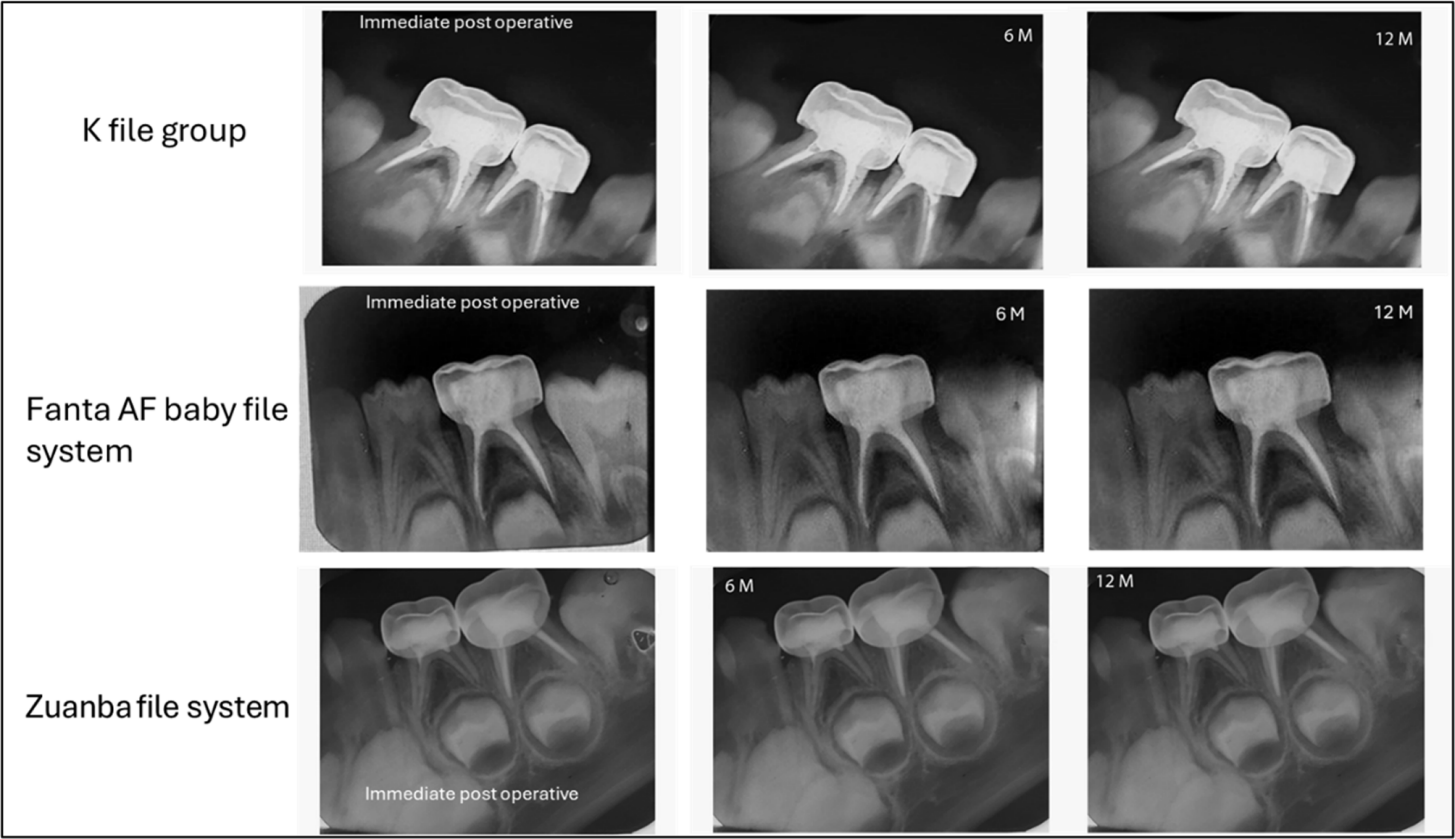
Radiographs of second primary molars treated with K-files, Fanta AF file system, or Zuanba file system at immediate post-operative, 6-month, and 12-month intervals
